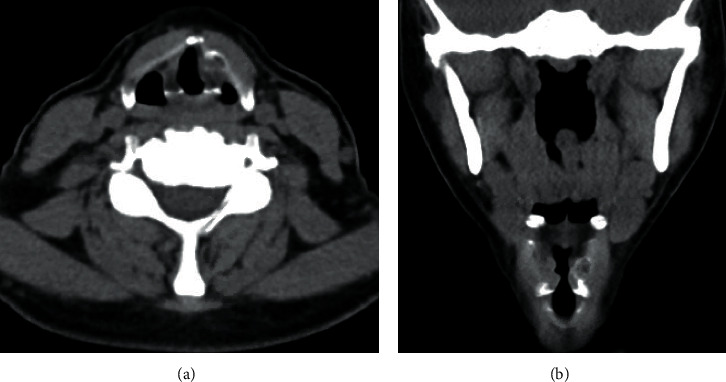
Axial (a) and coronal (b) CT without contrast demonstrating a mass arising from the left thyroid cartilage lamina with a thin calcified rim and a heterogeneous decreased attenuation center.
